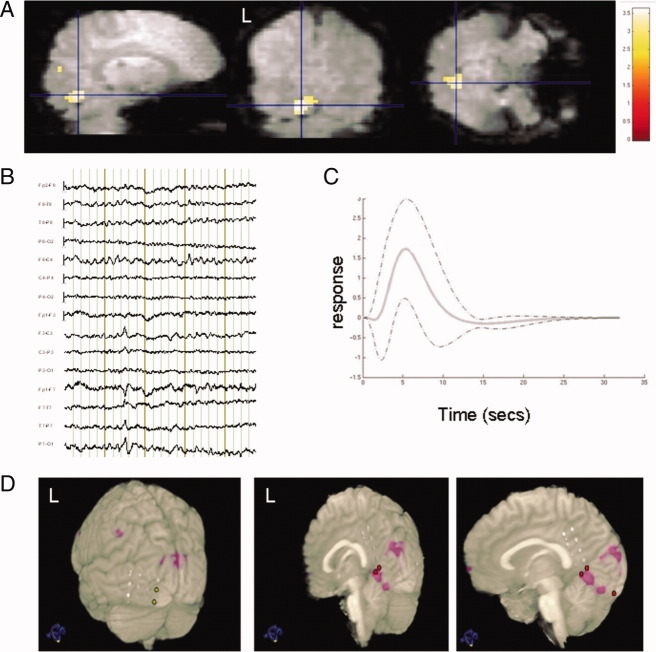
Results of electroencephalographic (EEG) functional magnetic resonance imaging (MRI) and intracranial EEG in a 21-year-old male patient (Patient 22) with normal structural MRI and focal epilepsy (age of onset, 9 years). EEG revealed left (L) frontotemporal spikes and sharp waves (maximal, F3–F7). Seizures consisted of a visual aura followed by a sensation of the eyes pulling to the right. The patient underwent intracranial recording with a 20-contact lateral occipital grid and 16-contact mesial occipital grid in addition to 2 × 6-contact occipital depth electrodes, following which he underwent surgical resection of the seizure onset zone, close to the left occipital pole, following which he was seizure free (International League Against Epilepsy scale outcome 1) 1 year after surgery. (A) Interictal discharge (IED)-correlated blood oxygen level-dependent signal (BOLD) signal change is overlaid on T2*-weighted echo planar imaging (SPM{F}: *z* = 3.87, *p* < 0.05 family-wise error corrected for multiple comparisons). (B) Scalp EEG shows events recorded during scanning. (C) The hemodynamic response related to the events in B is shown. (D) IED-related BOLD increase (magenta) overlaid (magenta) on a surface and volume rendering of patient T1-weighted MRI fused with computed tomography taken with intracranial electrodes in situ, showing the relationship of the BOLD change to the seizure onset zone (electrodes depicted in red), and irritative zone (depicted in yellow). The electrodes in the seizure onset zone are concordant with the cluster of BOLD increase containing the global statistical maximum. Note that volume rendered images show a cross section through the left medial occipital lobe. [Color figure can be viewed in the online issue, which is available at www.annalsofneurology.org.]
